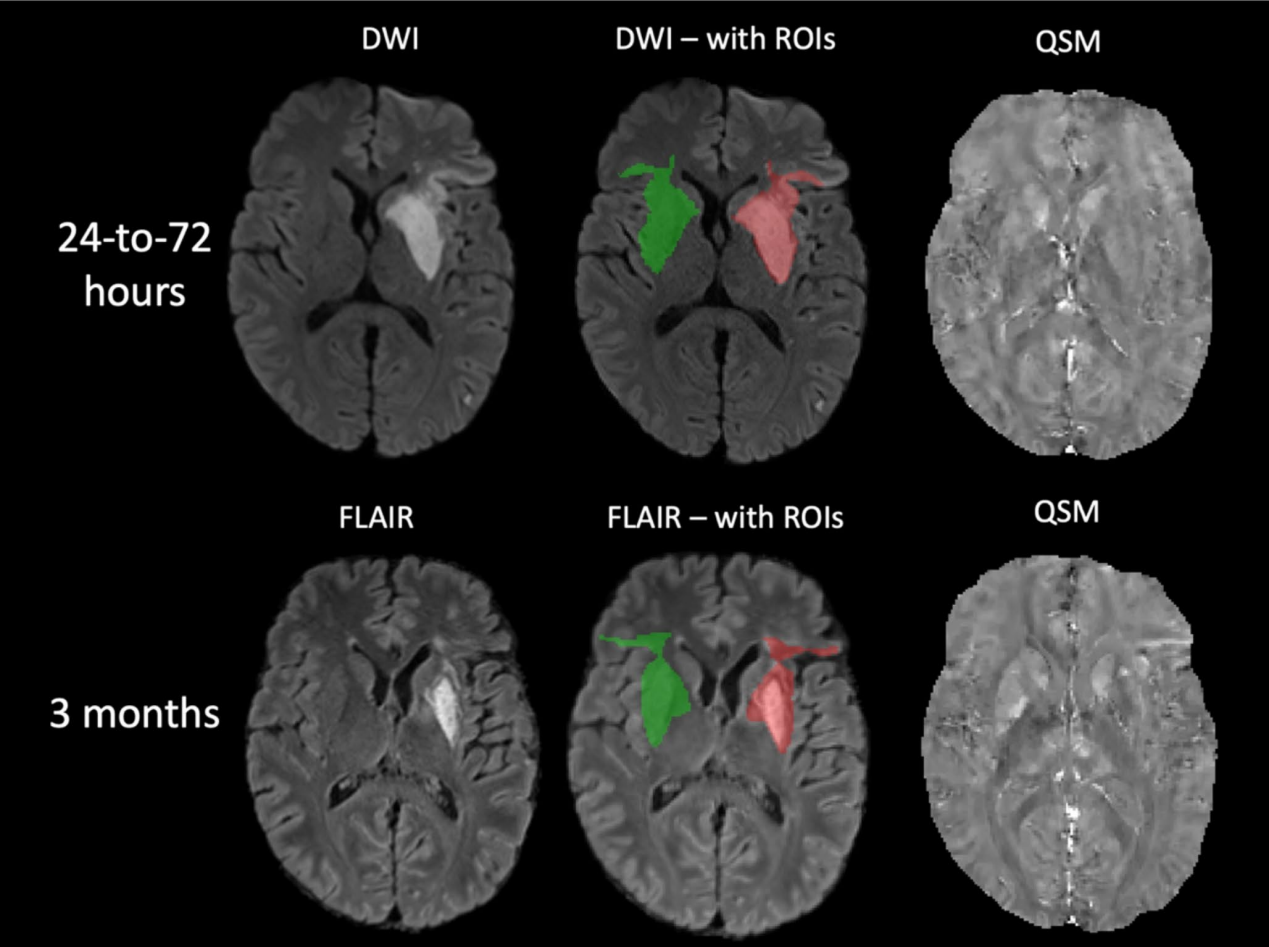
Representative DWI, FLAIR, and QSM images of a 55-year-old male with an M1 occlusion-type ischemic stroke. ROIs indicate the infarct (red) and the contralateral regions (green). DWI = diffusion-weighted imaging, FLAIR = fluid-attenuated inversion recovery, QSM = quantitative susceptibility mapping
